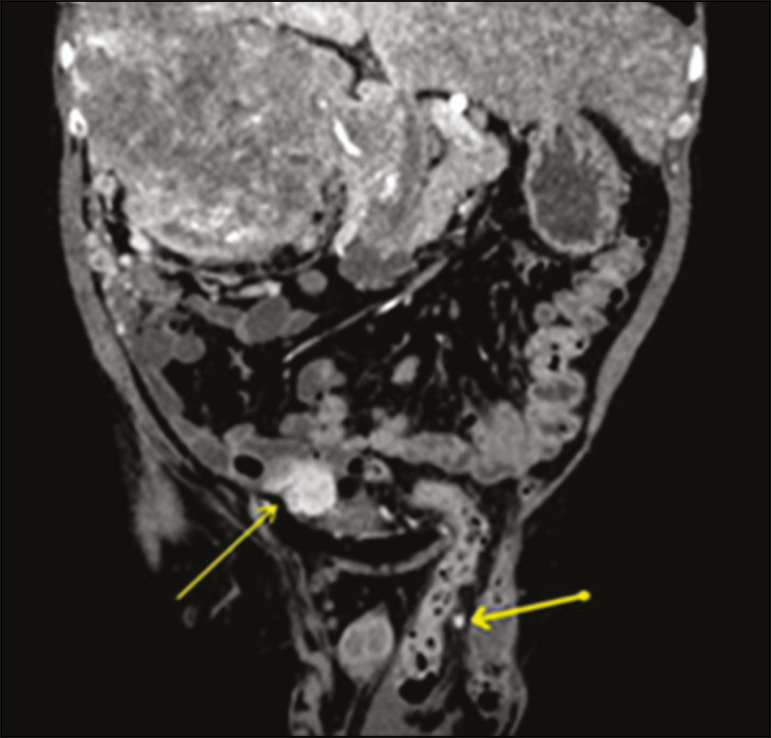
Coronal CT in the arterial phase showing a massive renal cell carcinoma that was hypervascularized on the right, together with nodules typical of peritoneal carcinomatosis, one of them in the left hernia sac (arrows).
